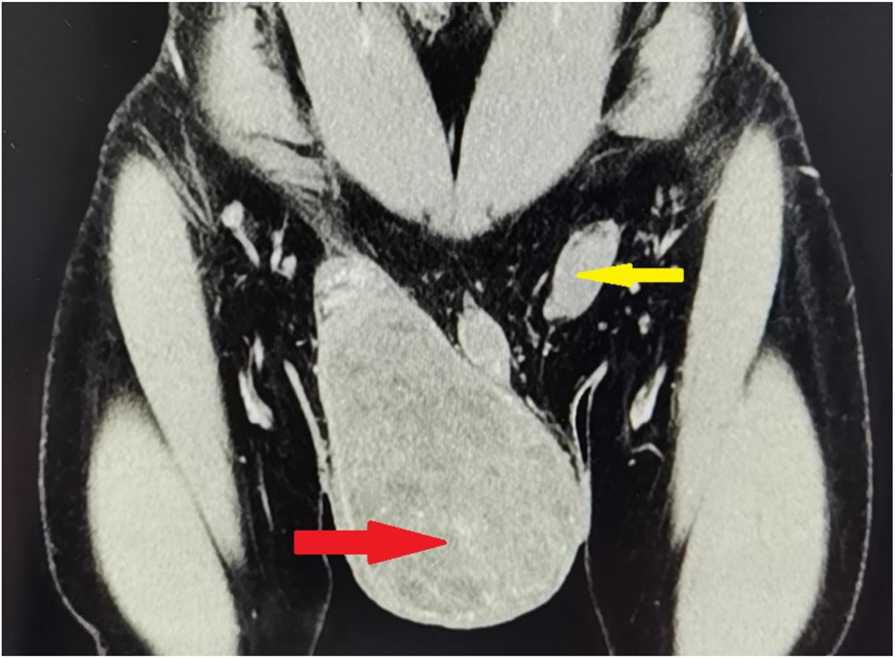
CT images of patients. The red arrow indicates the right testicular tumor, and the yellow arrow indicates the left cryptorchidism
